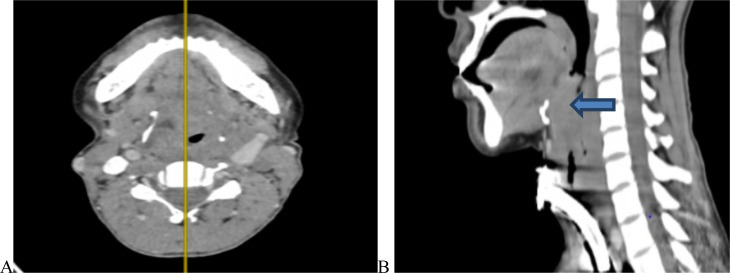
Post contrast CT findings of laryngeal cancer in 43 years old female patient, with heterogenous enhancement with oropharyngeal extension thyroid cartilage erosion(A) Transglottic pattern with hypopharyngeal extension (B)
